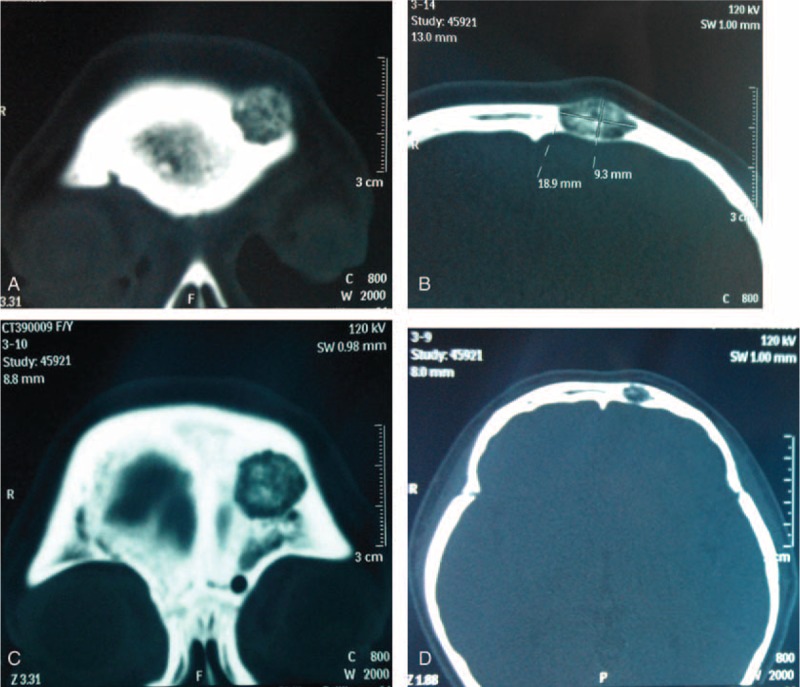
CT scan (bone window) demonstrated an intradiploic osteolytic mass.
